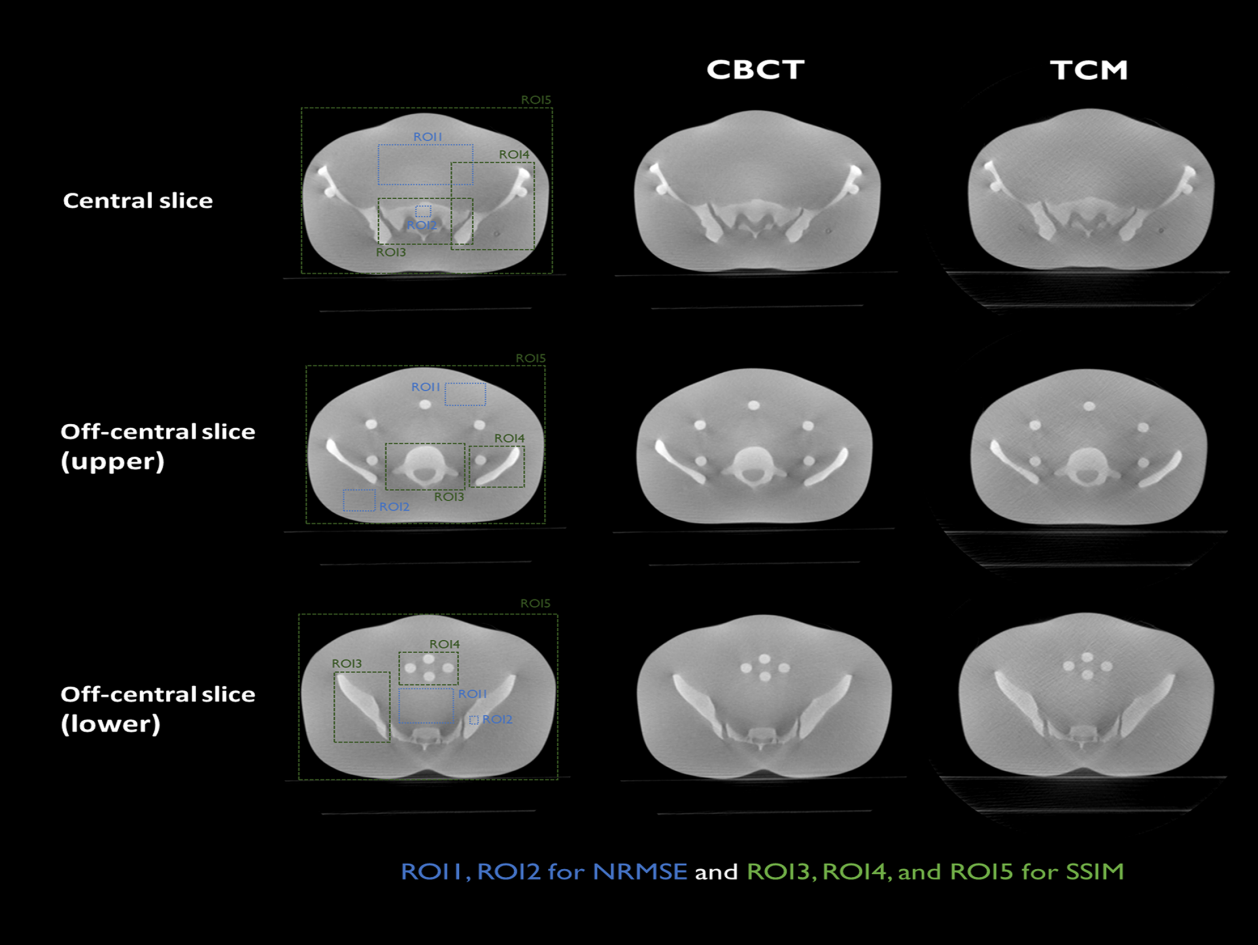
Reconstructed images of ATOM’s pelvic region from conventional CBCT and TCM methods. XVI images are from the central, upper, and lower off-center regions.
